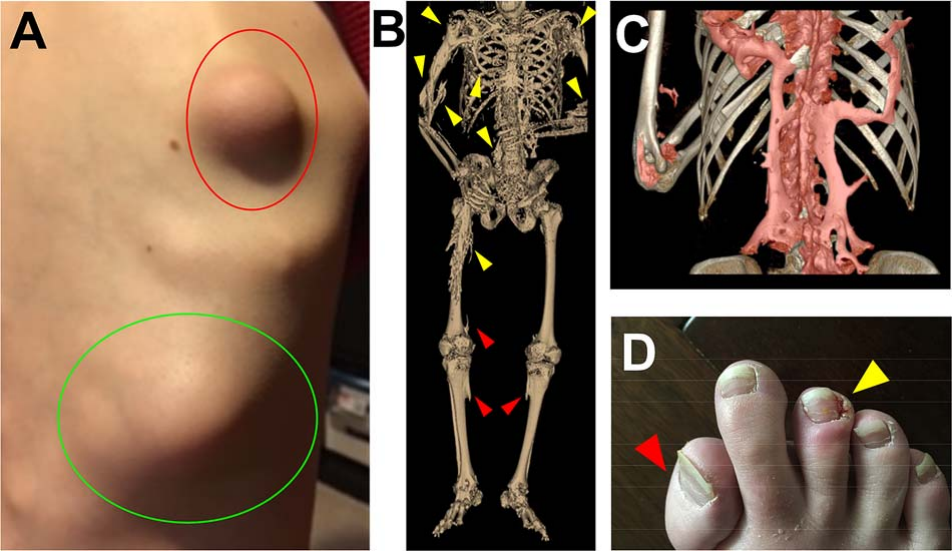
(A) Photograph of a right scapular/back flare, with erythema and induration (indicated by red circle), in a 9-year-old male patient with fibrodysplasia ossificans progressiva (FOP). This flare went on to restrict mobility in the right shoulder. A second older flare, with a different flatter morphology, is also identified (green circle). Image of the top flare was previously published and is reused here under the CC4.0 license.^44^ (B) Low dose whole body CT scan reconstruction is reproduced here^45^ without modification under the CC4.0 license except to add labeling. This 33-year-old male was in the natural history study. Representative osteochondromas are indicated by red arrows. Representative areas of heterotopic ossification (HO) formation are indicated by yellow arrows. (C) False colored thoracic CT scan reconstruction of a 37-year-old female patient with FOP showing accumulated HO (red) overlying the native skeleton. (D) Photograph of a 15-year-old female patient with FOP showing the great toe malformation (shortened, with malformed proximal metatarsal, classically seen in FOP; red arrow) and paronychia (yellow arrow), which can occur in patients taking palovarotene.
